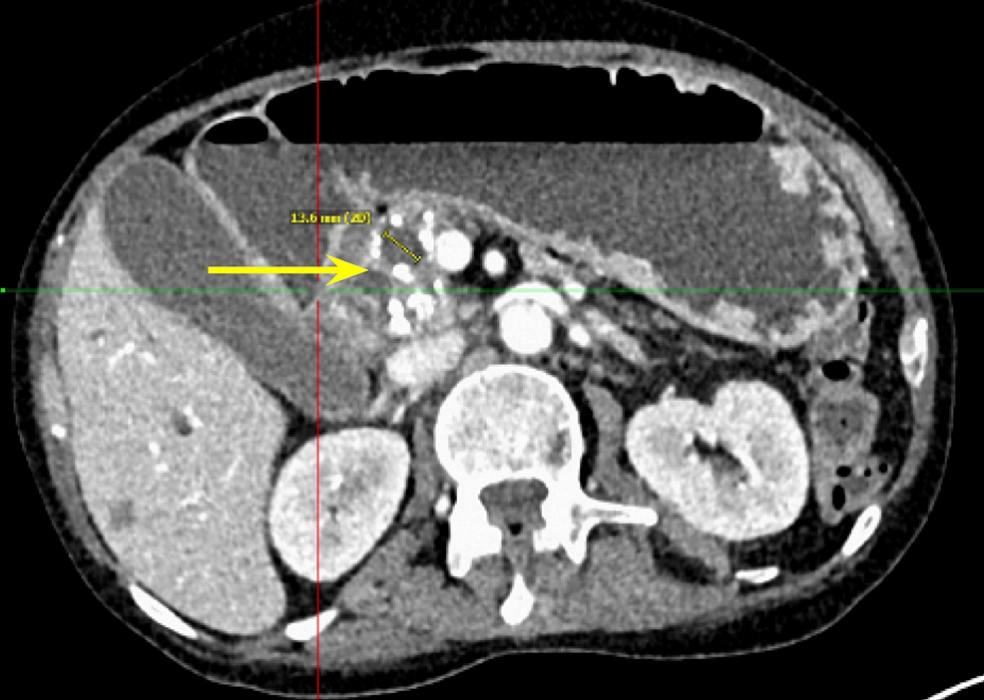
Computed tomography of the abdomen and pelvis showing dilation of the pancreatic duct, approximately 1.3 cm.
